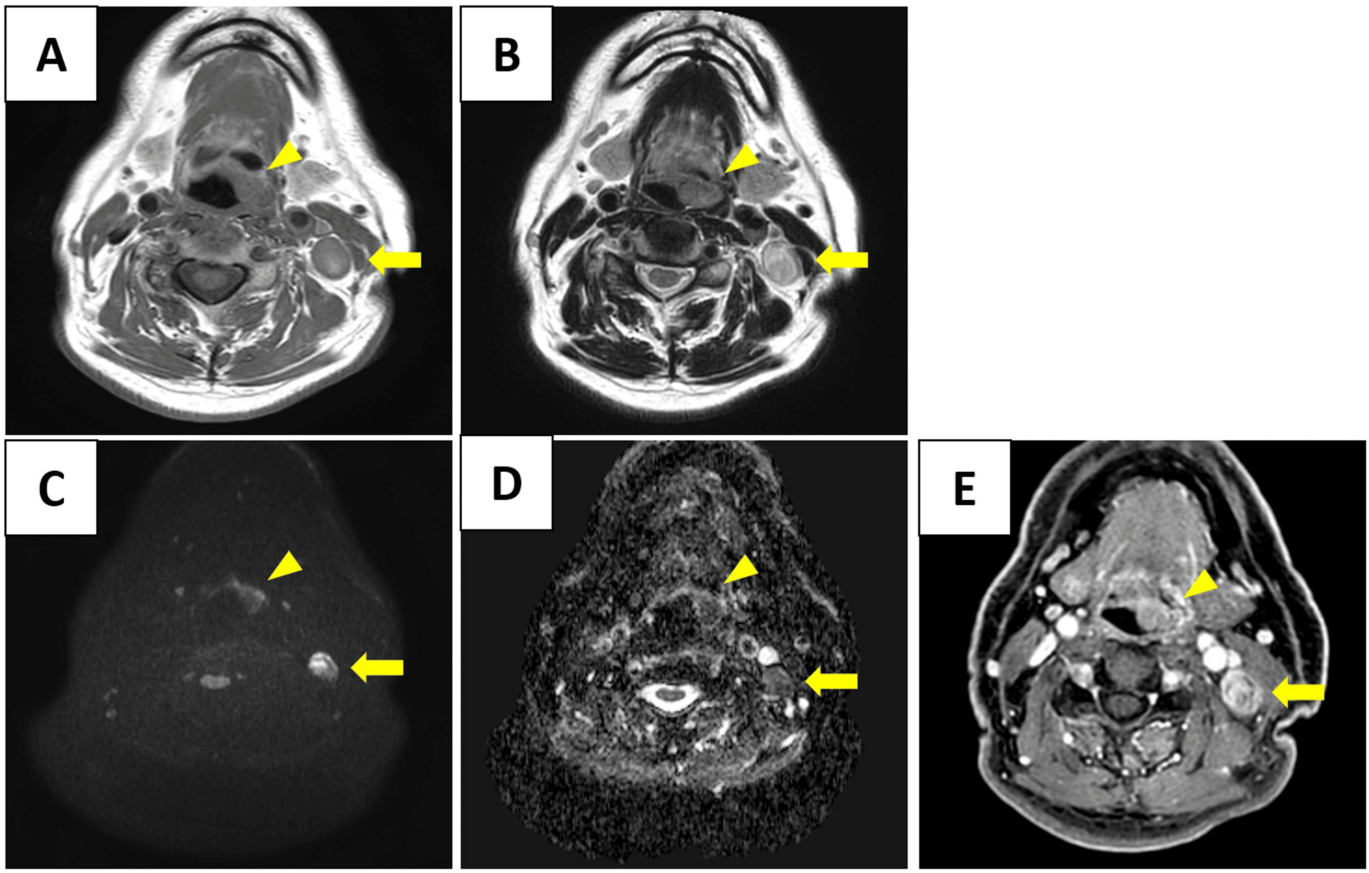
Magnetic resonance imaging (MRI) findings (all axial sections) (A) T1-weighted image, (B) T2-weighted image, (C) diffusion-weighted image (DWI), (D) apparent diffusion coefficient (ADC) map, (E) contrast-enhanced T1-weighted image with gadolinium. An irregular mass measuring 18 × 12 mm is observed on the left side of the epiglottis, showing iso-intensity on both T1- and T2-weighted images (yellow arrowheads). Enlarged lymph nodes are also noted in the left internal jugular region (yellow arrows). The epiglottic tumor and cervical lymph nodes appear hyperintense on DWI and hypointense on the ADC map, indicating restricted diffusion. Both lesions exhibit contrast enhancement following gadolinium administration. Similar to CT, these MRI findings are not pathognomonic and could overlap with those of more common supraglottic malignancies such as squamous cell carcinoma, underscoring the need for tissue diagnosis.
